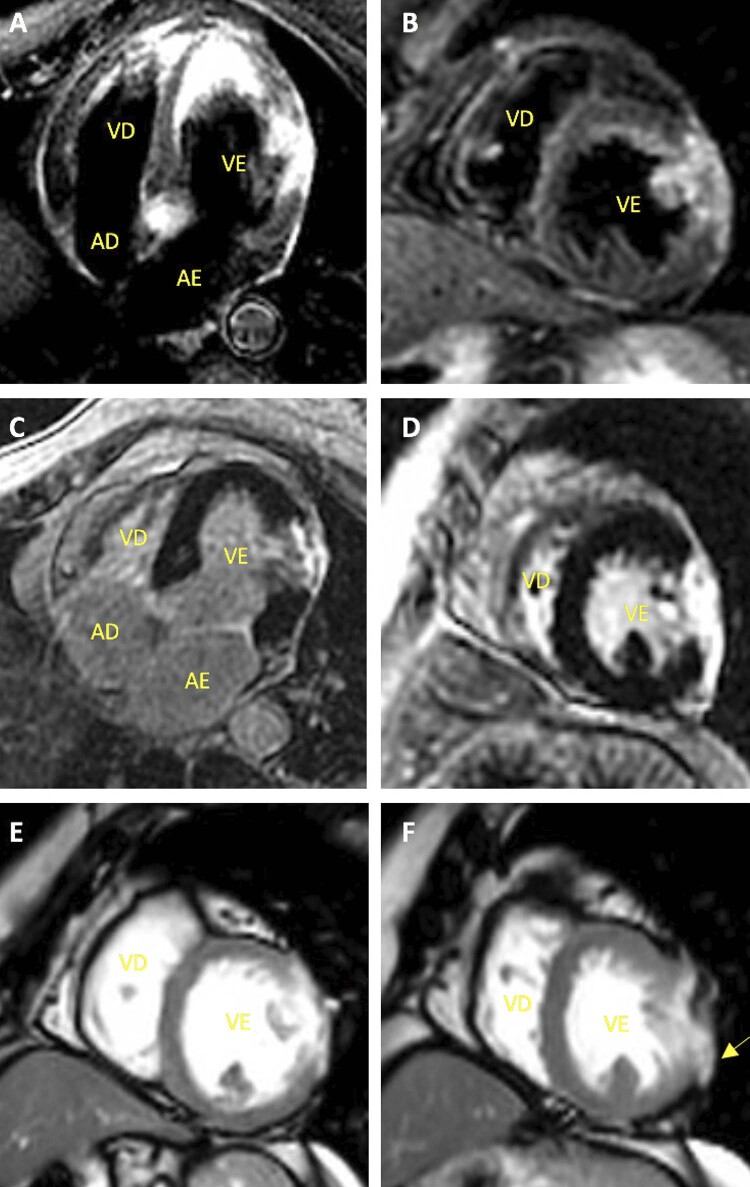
Ressonância magnética cardíaca. (A) Imagem de quatro câmaras e (B) Imagem de eixo curto, sequências de recuperação de inversão de tau curta ponderadas em T2 (T2-weighted short-tau inversion recovery images, STIR), mostrando sinal hiperintenso transmural do segmento médio da parede lateral compatível com edema; (C) Imagem de quatro câmaras e (D) Imagem de eixo curto, sequências de realce tardio miocárdico com gadolínio, mostrando realce transmural do mesmo segmento, sugerindo necrose miocárdica; (E) fase telediastólica e (F) fase telesistólica, sequências de cine obtidas por precessão livre no estado estacionário (steady-state free precession, SSFP), mostrando discinesia do segmento médio da parede lateral e uma protuberância sacular sugerindo pseudoaneurisma (seta amarela).VE: ventrículo esquerdo; AE: átrio esquerdo; VD: ventrículo direito; AD: átrio direito.
